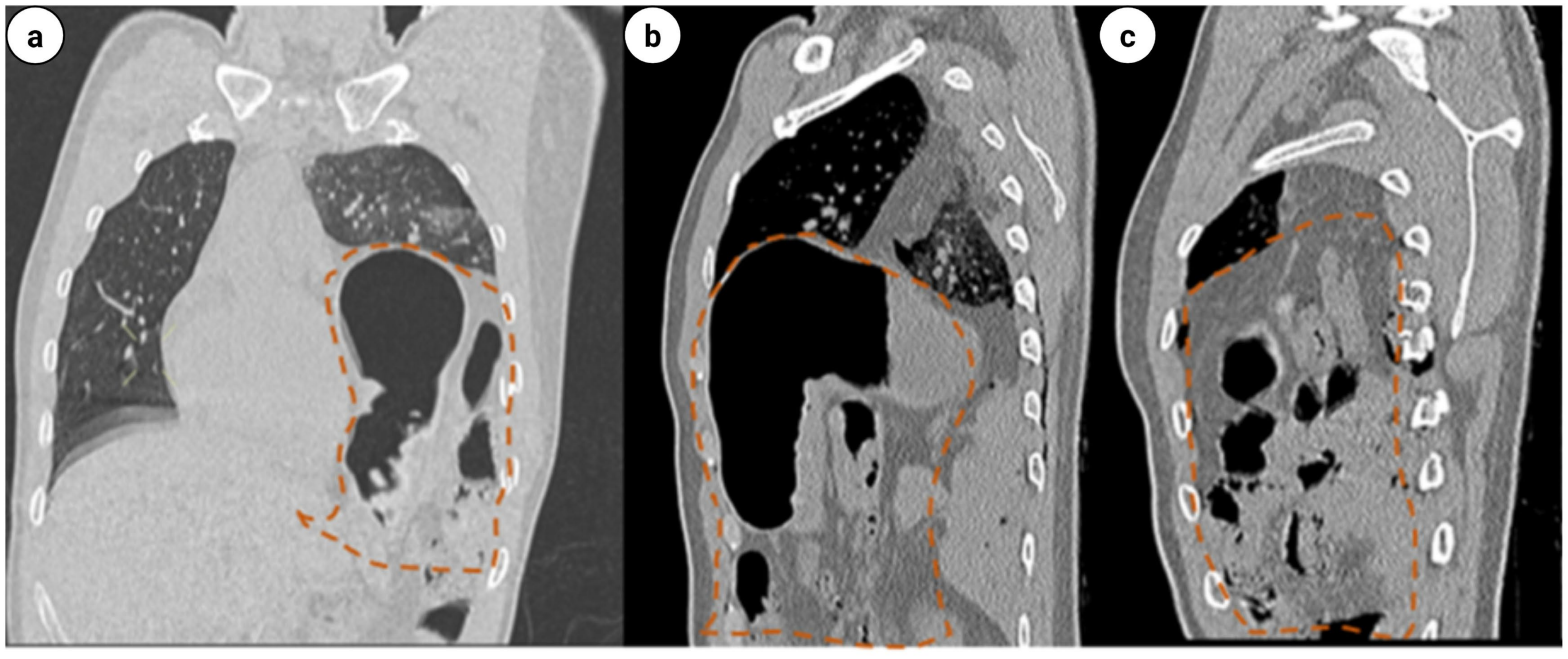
(a) Coronal and sagittal CT scans of the chest: left diaphragmatic rupture complicated with left diaphragmatic hernia. (b,c) The gastric fundus, gastric body segment, portion of small intestine, and transverse colon were identified herniating into the left thoracic cavity (within the red dashed line).
